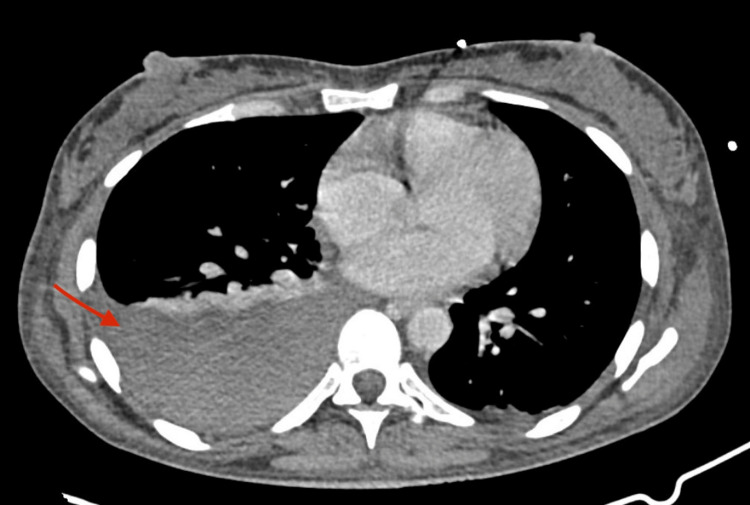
Thoracic CT scan (axial plane) demonstrating the hemothorax (red arrow)
